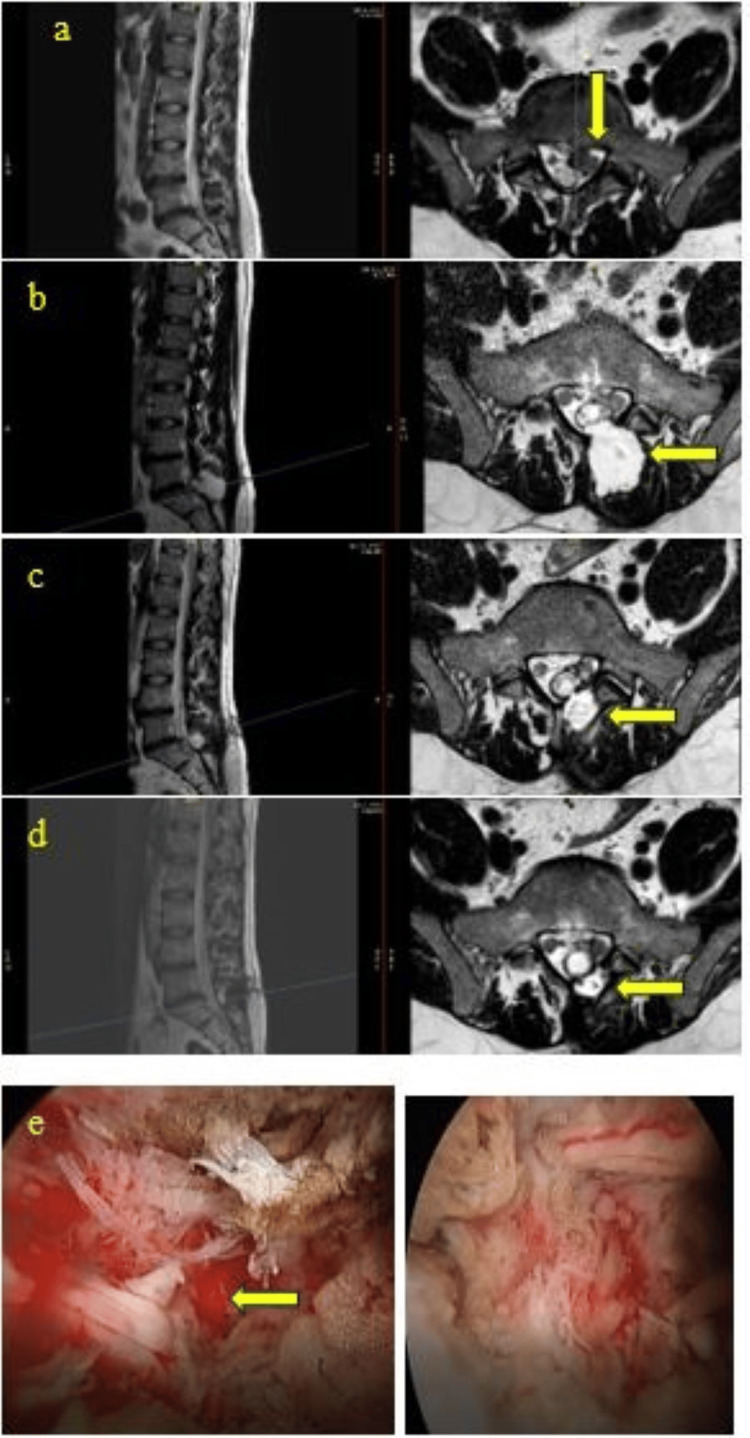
Dural Tears and Repeated Surgeries (a) Preoperative axial and sagittal T2 MRI of the left L5/S1 disc herniation compressing the nerve root (yellow arrow). (b) Postoperative axial and sagittal T2 MRI showing a large pseudomeningocele (yellow arrow). (c) Axial and sagittal T2 MRI following the primary surgical dural repair showing a decrease in pseudomeningocele but an incomplete repair with CSF collection (yellow arrow). (d) Axial and sagittal T2 MRI after the second surgical repair showing a good and successful repair (yellow arrow). (e) Intraoperative images showing a significant durotomy, with nerve roots seen outside the dural sac (yellow arrow) CSF: cerebrospinal fluid
